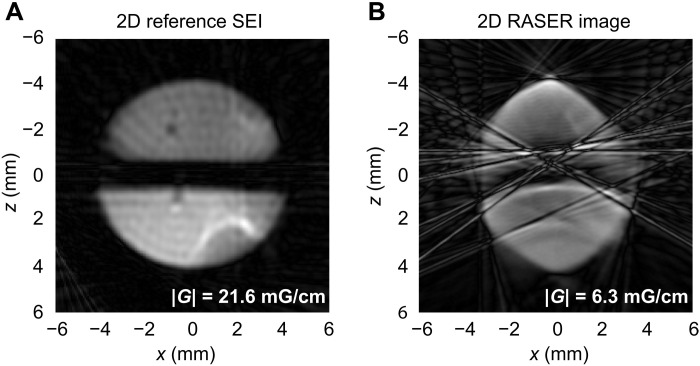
2D SEI and 2D RASER image. (A) 2D SEI and (B) 2D RASER MRI measured at 3.9 mT. The 2D images (A and B) are obtained by projection reconstruction of 30 projections each. These 1D projections are measured with the sequence in Fig. 2 (C and D) from different angles by varying *G_x_* and *G_z_* such that *G_x_*^2^ + *G_z_*^2^ = constant. In (A), the two capillaries used for *p*-H_2_ supply are visible around *x* = −1 mm, *z* = 0.5 mm and *x* = −1.5 mm, *z* = −2 mm for each chamber. The RASER image (B) is recorded at a 3.5 times smaller gradient than (A), but both spatial resolutions are similar. The RASER image is affected by interference lines. The origin of these artifacts is discussed in the text and in section S5.
